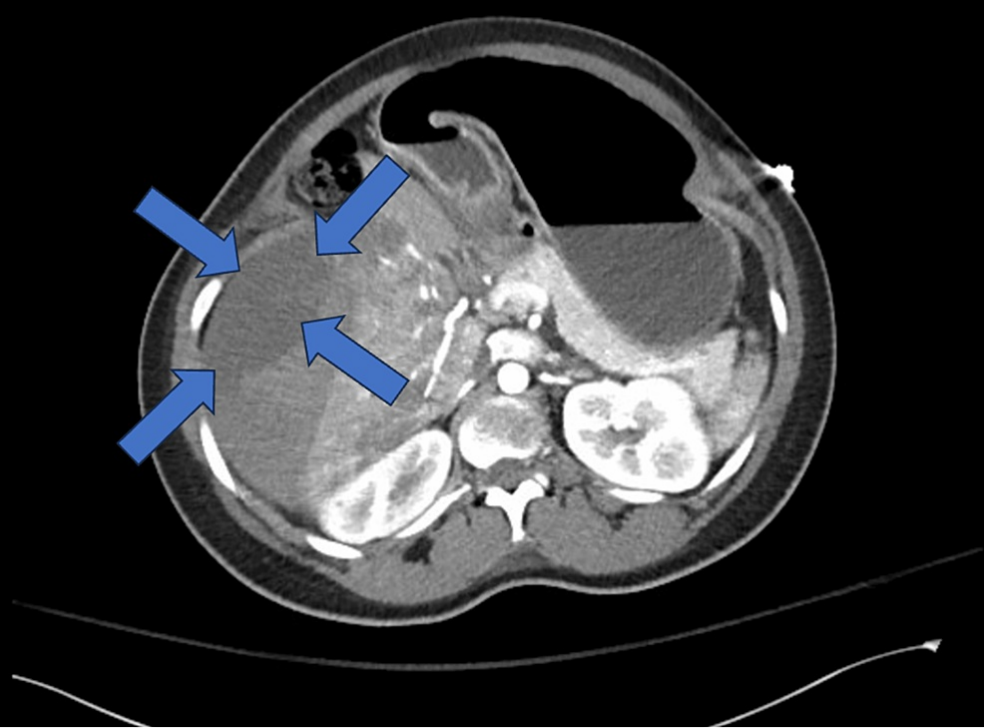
CT scan of abdomen and pelvis The blue arrows highlight the edges of the subcapsular hematoma CT: computed tomography
